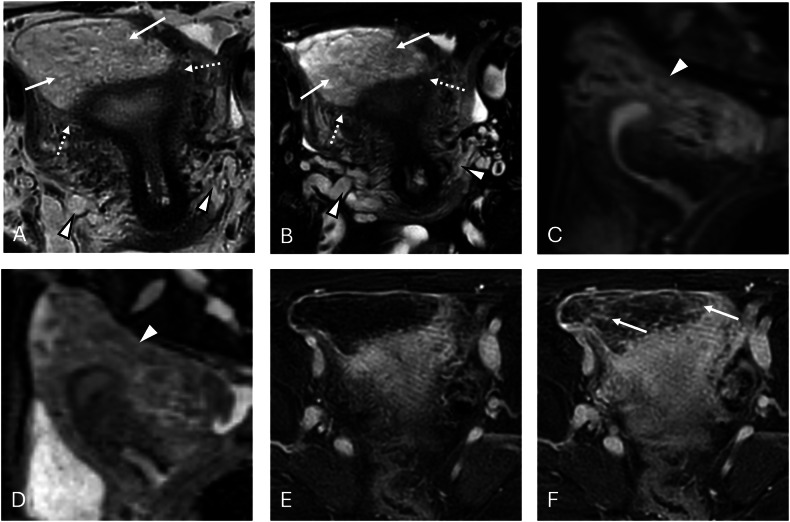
Gynaecological venous malformation (GVM) confirmed by uterine biopsy in a 34-year-old patient and after a successful spontaneous pregnancy. A Axial T2-W and B T2-W fat-suppressed MR images show a well-defined, T2-hyperintense fundal lesion with a sponge-like appearance (arrows), sharply demarcated from the adjacent normal myometrium (dotted arrows). Parametrial venous varicosities are present bilaterally (arrowheads), a finding that may be associated with GVM but is non-specific; in this patient, these were not present on pre-pregnancy MRI, suggesting venous insufficiency. C Sagittal diffusion-weighted imaging (DWI) and D apparent diffusion coefficient (ADC) map MR images show no diffusion restriction (arrowheads). E Axial dynamic post-contrast T1-W fat-suppressed MR images at arterial and F at venous phase show slow and progressive peripheral enhancement of the lesion (arrows) relative to adjacent myometrium, without early enhancement, nidus, or solid tissue component, supporting slow venous flow
